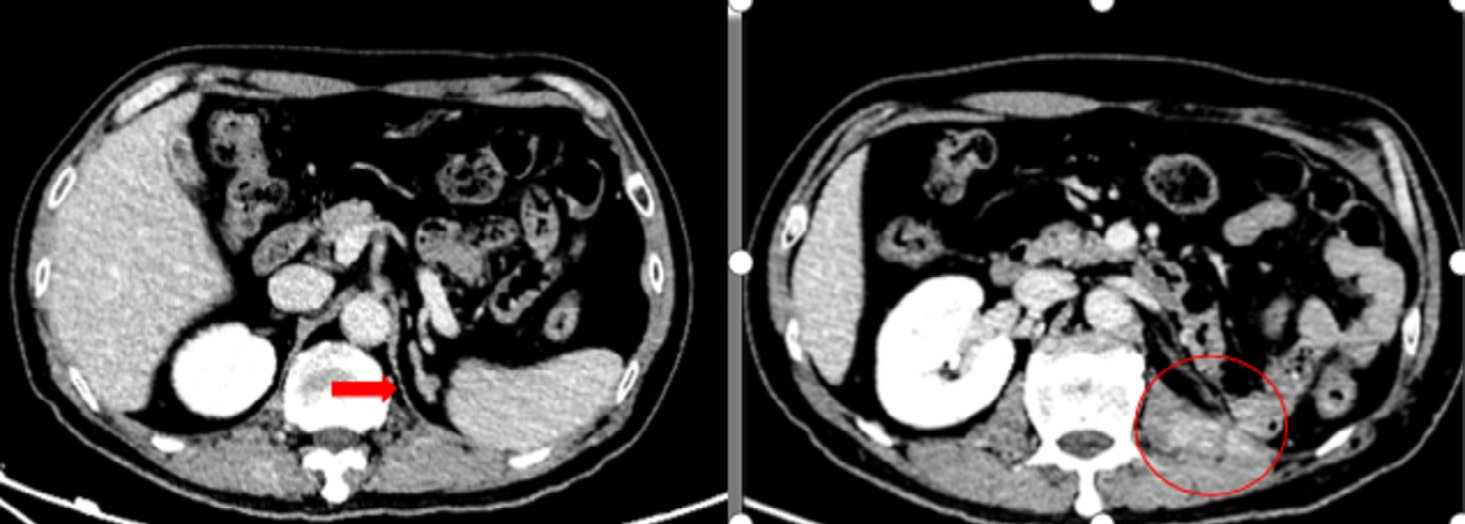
Urinary CT in October 2017.
